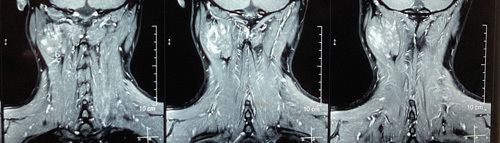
MRI of the cervical spine; T1-weighted scan (coronal section) showing intramuscular hemangioma of splenius capitis.
